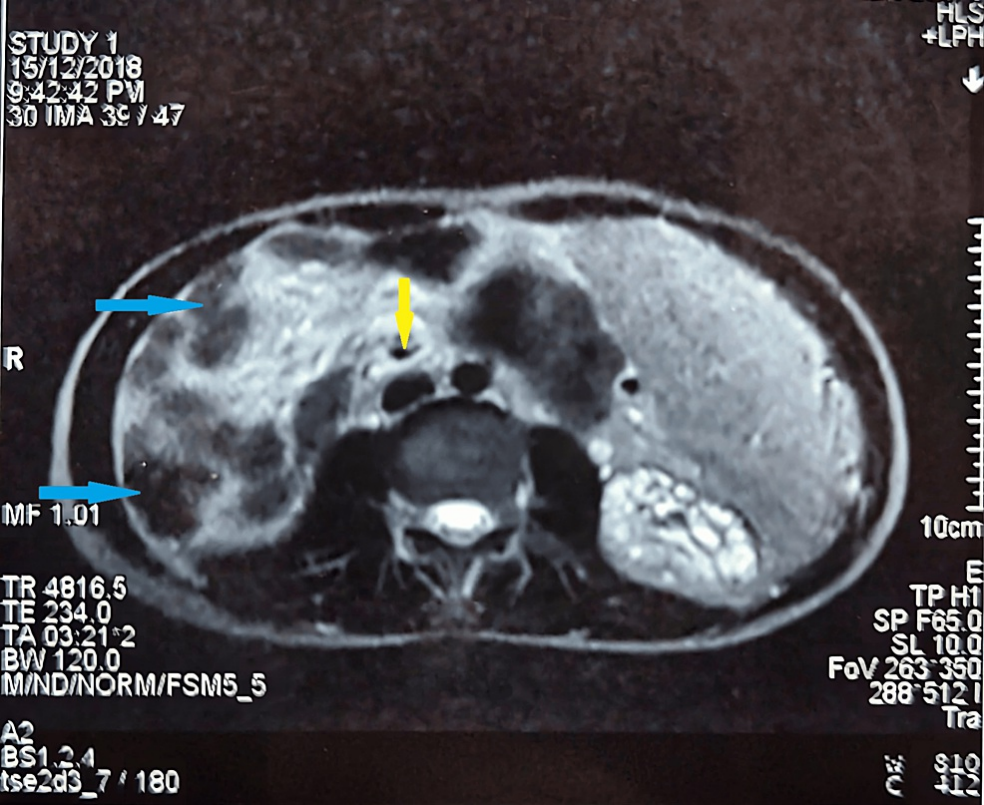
Magnetic resonance imaging showing hepatomegaly with cystic dilatations (blue arrows) and biliary ectasia (yellow arrow).
